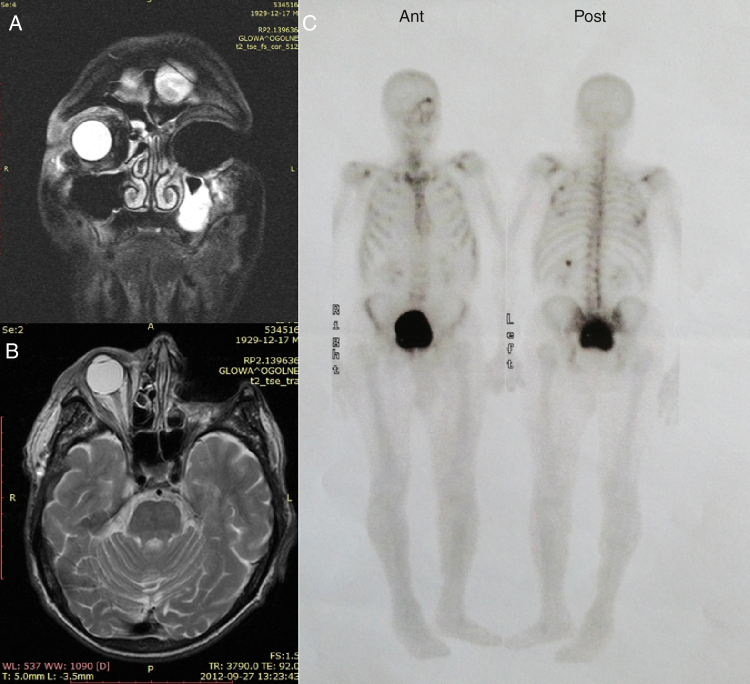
Magnetic resonance imaging scan: left orbit after exenteration without pathologic enhancement, mucus fluid in left maxillary sinus-frontal and plain projection (A and B). Bone scintigraphy, multiple intense enhancement in left orbit, and bony skeleton due to metabolic and postoperative changes (C).
